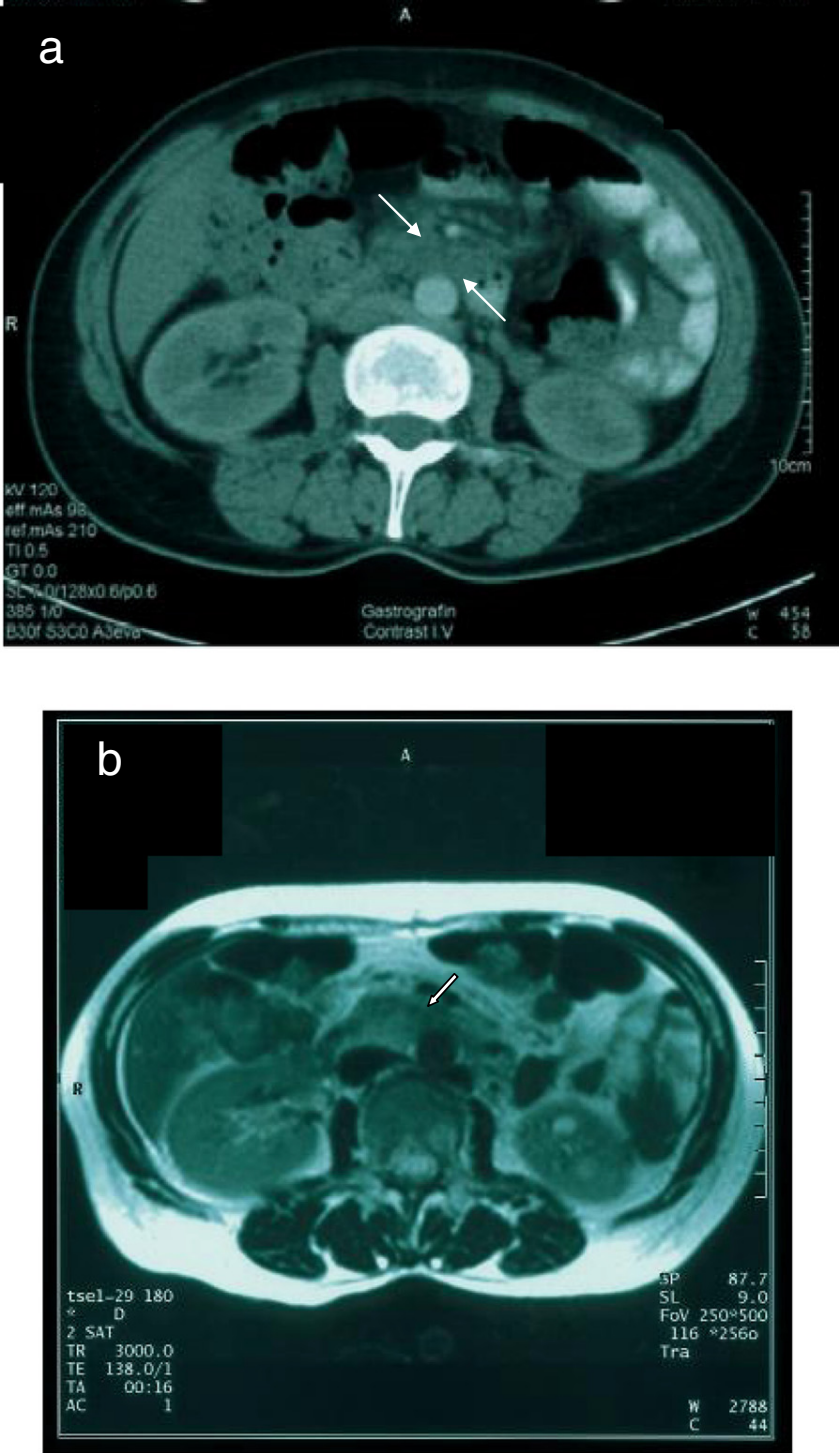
(a) Computed tomography images show presence of a low-attenuation mass engulfing the anterior surface of the infrarenal aorta (arrows). Note there is no apparent soft-tissue enhancement of the lesion. (b) Axial T2-weighted magnetic resonance image shows low to intermediate signal of the mass (arrow).
